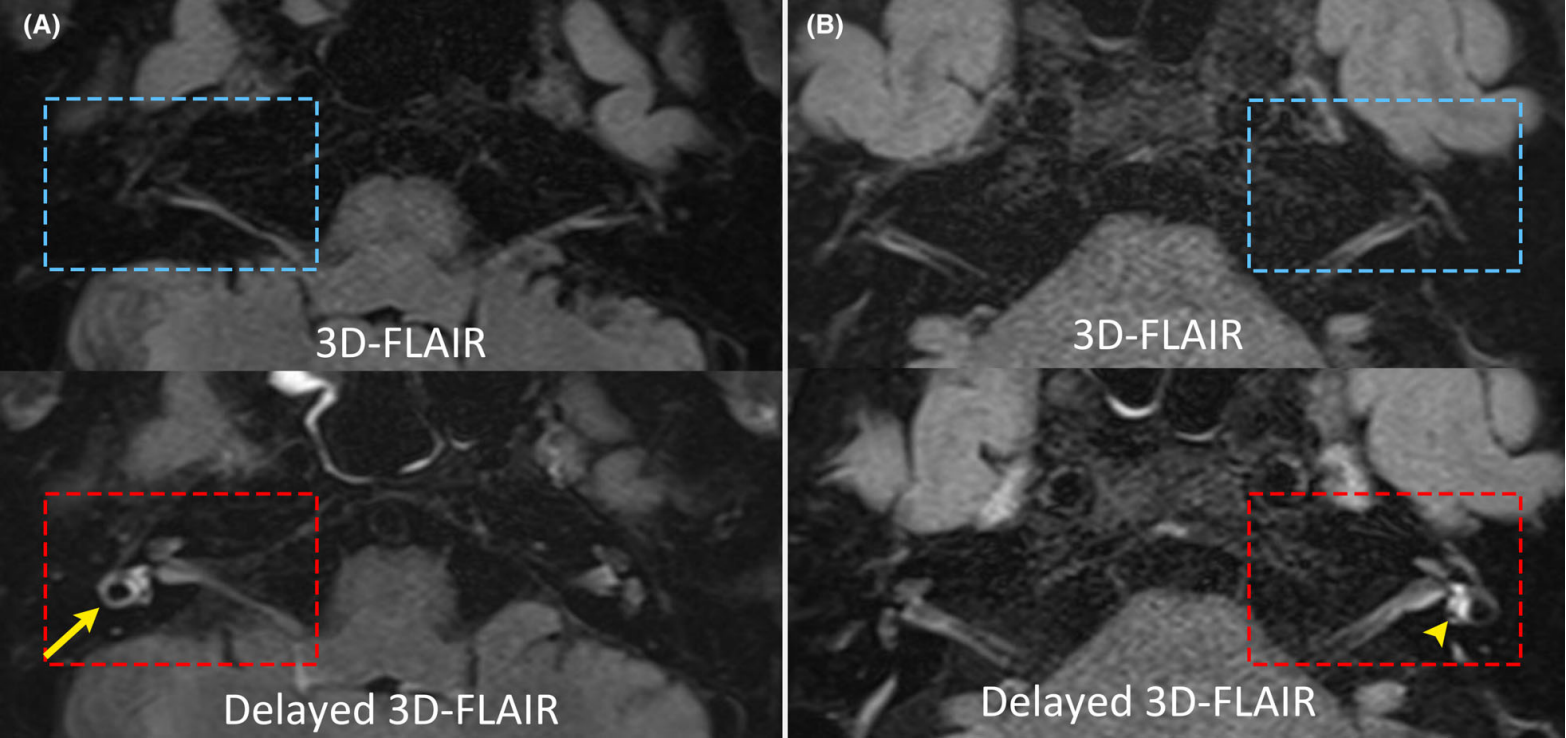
Representative MRIs in patients with acute unilateral peripheral vestibulopathy/vestibular neuritis. (A) No signal changes are present on baseline 3D‐FLAIR image in a patient with right acute unilateral peripheral vestibulopathy/vestibular neuritis (upper row). Contrastingly, a robust gadolinium enhancement is observed in the horizontal canal (arrow) and vestibule on the 4‐hour‐delayed 3D‐FLAIR image (red rectangle; lower row). (B) gadolinium enhancement is found in the vestibule (arrowhead) on the 4‐hour‐delayed 3D‐FLAIR image (lower row) while showing no discernible changes on baseline 3D‐FLAIR image (upper row).
